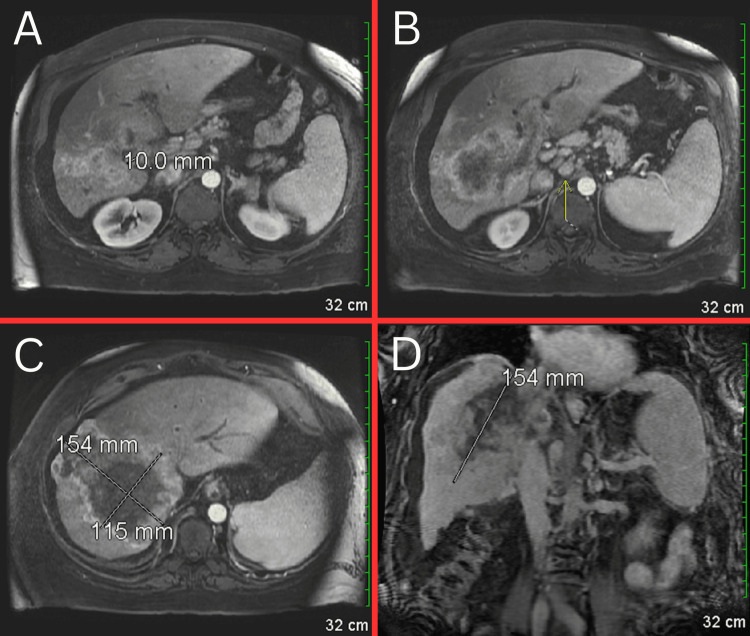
MRI showing ascites around the liver and spleen, as well as trace bilateral pleural effusions. The gallbladder appears markedly abnormal, possibly containing sludge or stones, with some enhancement of the gallbladder wall (Figure 6A). Numerous mildly enlarged nodes (yellow arrow) are noted in the porta hepatis, with the largest measuring 1.5 x 2.0 cm (Figure 6B). A large mass measuring 11.5 x 15 x 15 cm is present, involving a significant portion of the right lobe of the liver (Figure 6C and Figure 6D). The mass extends superiorly through the liver capsule beneath the diaphragm and involves the anterior and posterior segments of the right hepatic lobe, extending into the caudate lobe. MRI: magnetic resonance imaging
